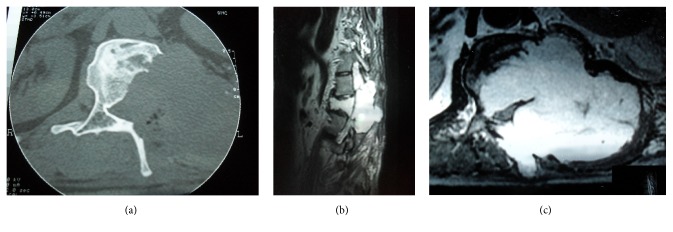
(a) CT: the destruction of the L1 vertebra, compared to Figure 2(b), has obviously become more important. (b) MRI, sagittal T_2_WI: the kyphotic deformity is evidently more prominent. (c) MRI, axial T_1_WI, with gadolinium: the dimensions of the hemangioma have clearly increased; the destruction of the L1 vertebra has obviously become more important.
